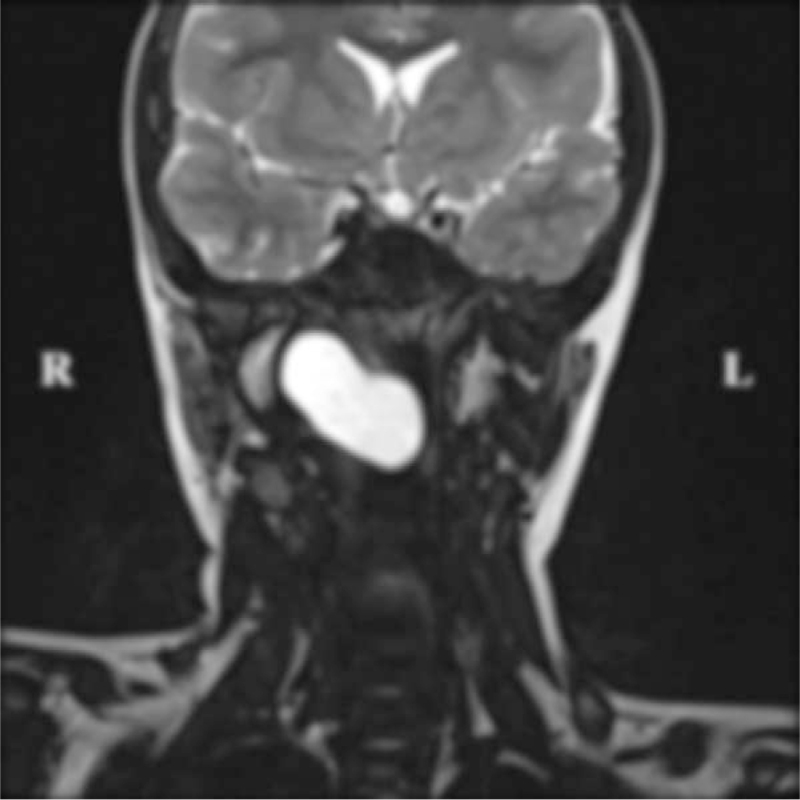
Preoperative plain and enhanced MRI of the nasopharynx display that the right parapharyngeal space extends to the nasopharyngeal cavity, there is a cystic isolow signal intensity on T1WI and high signal intensity on T2WI, and fat suppression shows high signal intensity on T2WI.
